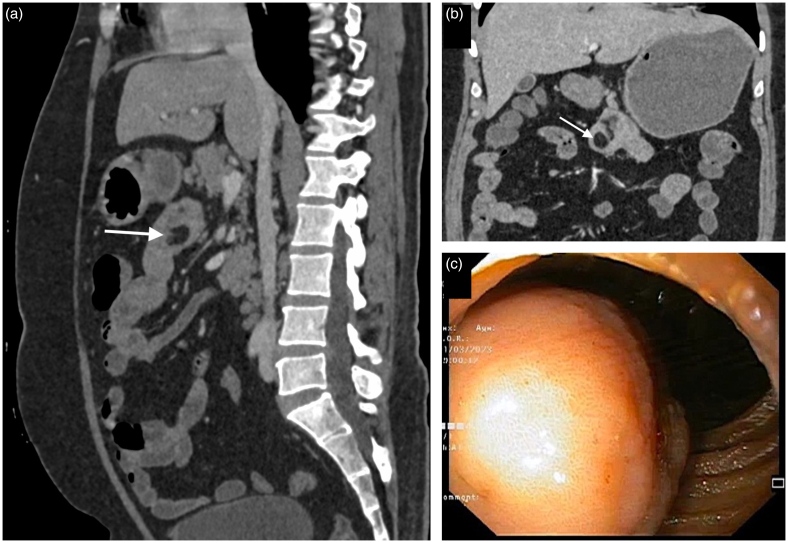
(a and b) Sagittal and coronal sections of abdominal CT scan showing fat-attenuating lesion in duodenum. (c) Endoscopic view showing a smooth submucosal lesion obliterating the duodenal lumen.
